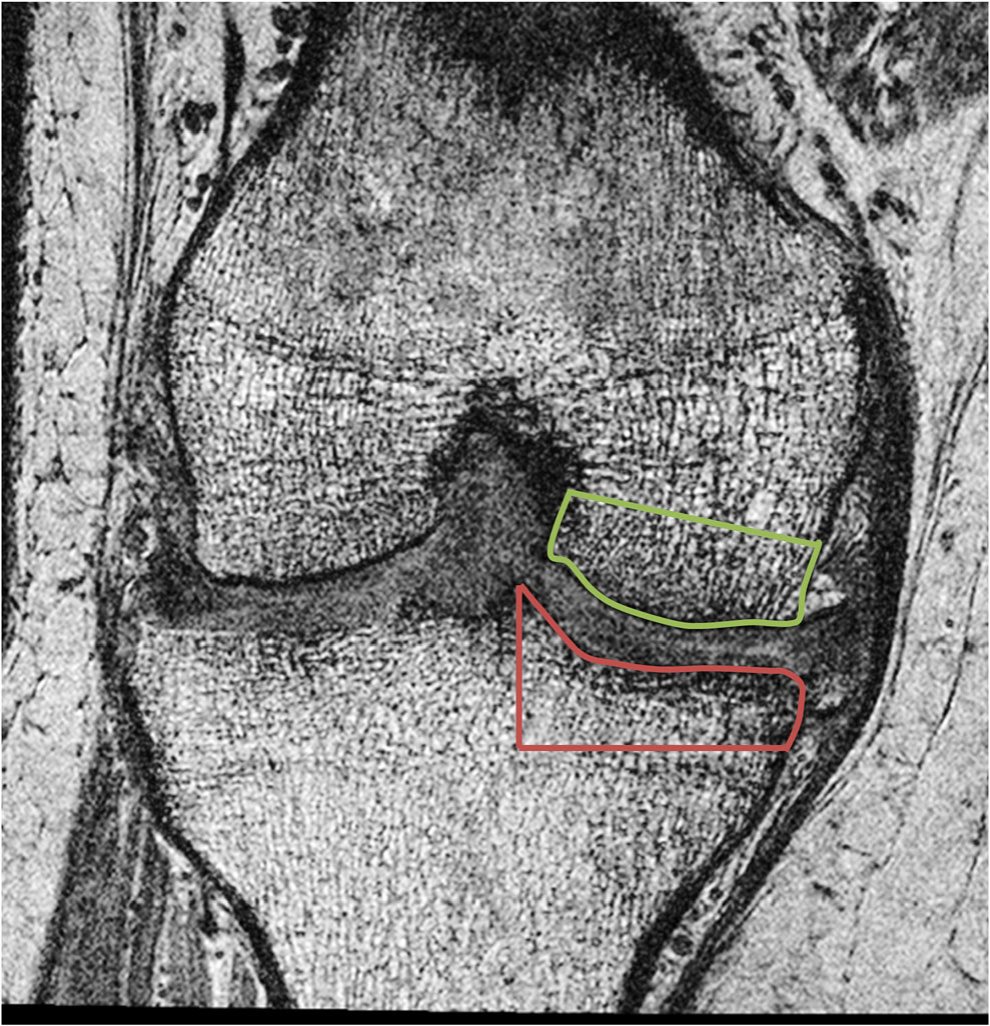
Example coronal-oblique 3D FISP MR image demonstrating ROI placement in the medial tibial (red) and medial femoral (green) subchondral bone
